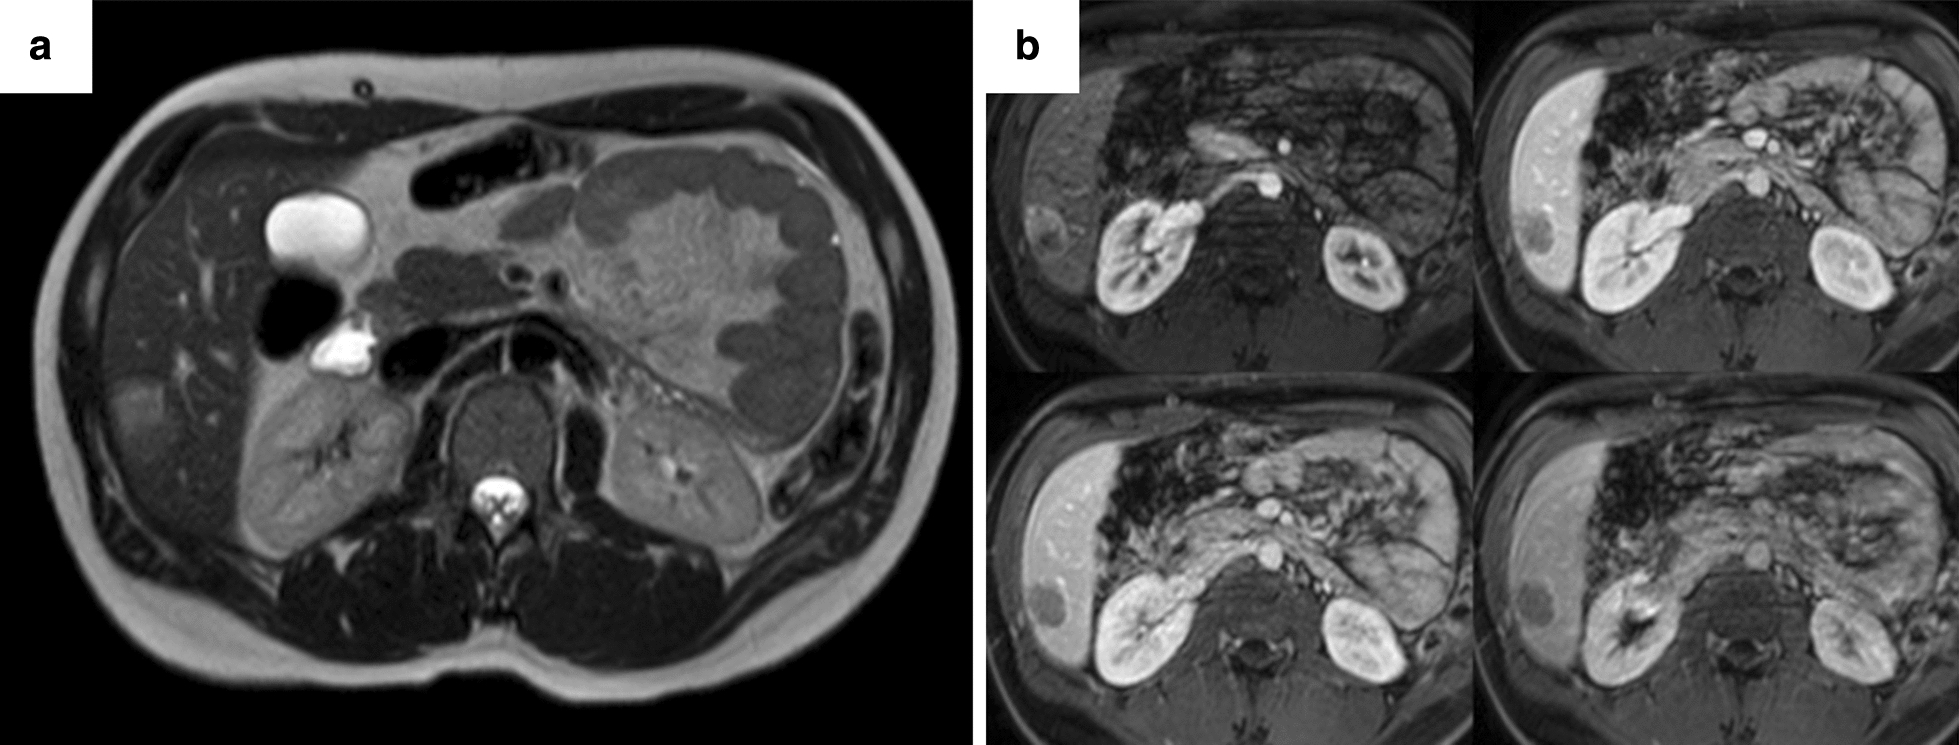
a, b MRI scans with intravenous contrast. Focal liver injury at segment 6 of 3 × 2.2 cm, hypointense at T1, and discrete hyperintense at T2 with diffusion restriction (a). In the dynamic study, it presented enhancement in the arterial phase with washing in the rest of the phases, with a more hypovascular area afterwards (b)
